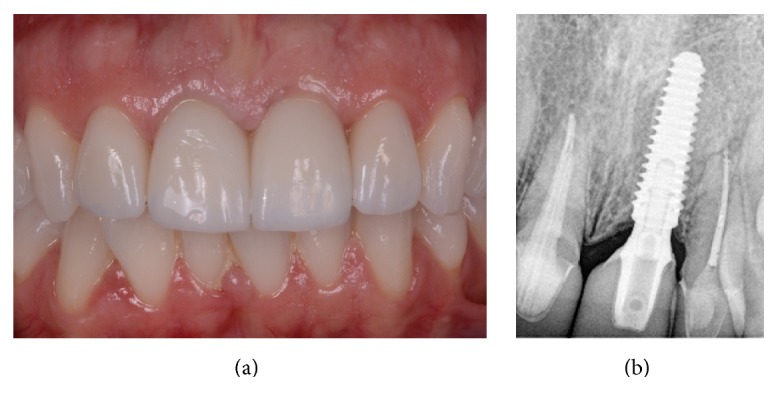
The definitive ceramic crown 1 year after the delivery. (a) An aesthetically pleasing result has been maintained clinically, and the patient is fully satisfied; (b) the radiographic control confirms the stability of the hard tissues around the implant.
